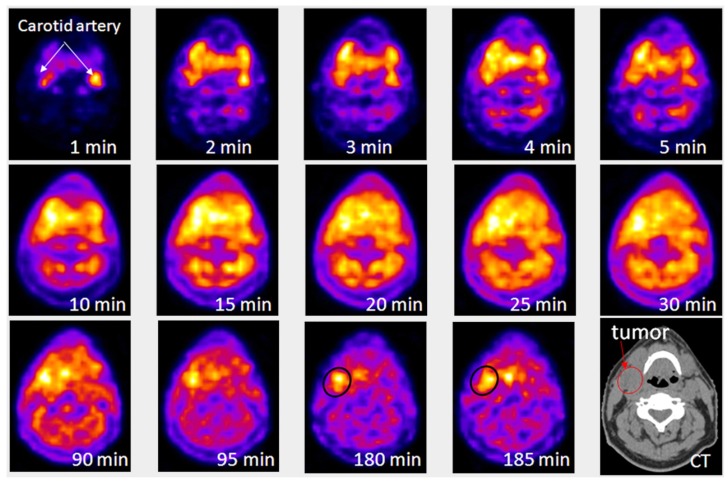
Results of a dynamic Positron Emission Tomography (PET) scan as a function of time after injection with ^18^F-fluoromisonidazole. A single reconstructed PET slice is displayed through the center of the head and neck tumor. First 5 frames were of 1-min duration, followed by 5 frames of 5-min duration. At 30 min after injection, the patient was removed from the scanner and then reimaged at 90 and 180 min after injection. Images were co-registered using a low-dose CT scan (depicted in the final image in the series). This series shows evolution of ^18^F-fluoromisonidazole distribution within the patient from initial blood pool to selective sequestration within the hypoxic tumor subvolume. This research was originally published in JNM. Carlin, Sean, and John L. Humm. PET of hypoxia: current and future perspectives. J. Nucl. Med. 2012, 53, (8), 1171–1174. © by the Society of Nuclear Medicine and Molecular Imaging, Inc. [26].
